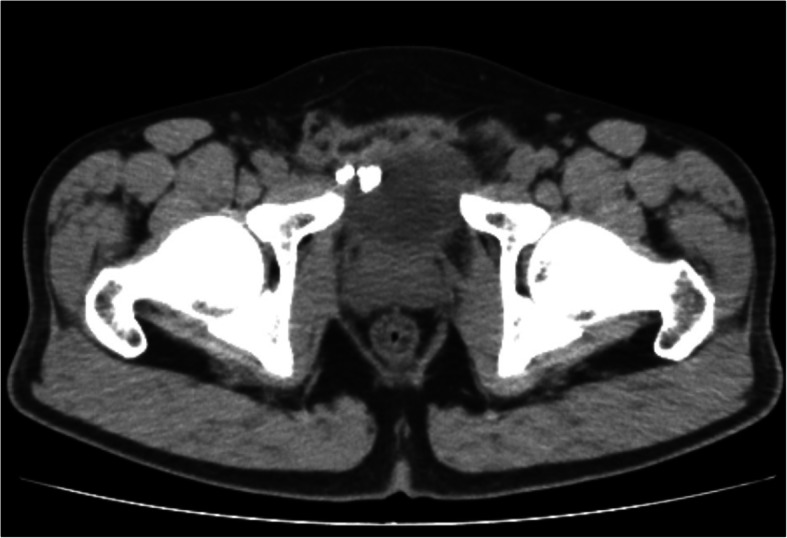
Abdominal plain CT-scan showing bladder stones and calcified tissue around the right inguinal region
